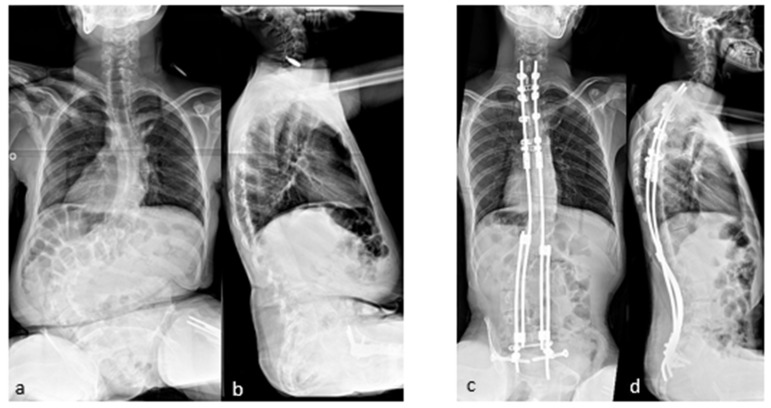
(a–d) Pre- and postoperative radiographs of 14-year-old patient with cerebral palsy who underwent surgery with a reinforced lumbar four-rod construct.
